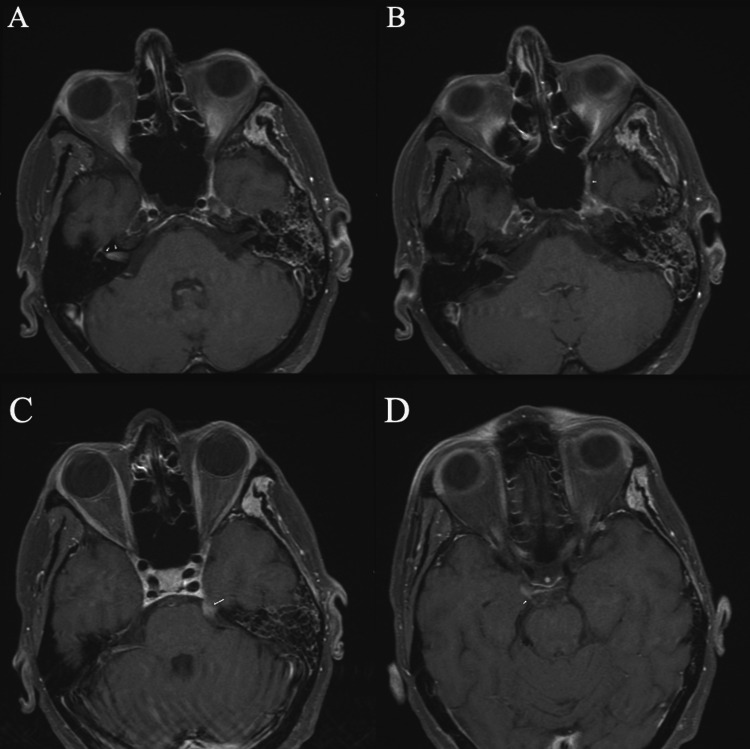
MRI of the brain with contrast revealing enhancement in multiple cranial nerves. (A) Linear post-contrast enhancement (*see arrows*) involving the right seventh and eighth nerve complex within the internal auditory canal, the basal turn of the right cochlea, and discontinuous enhancement of the right seventh cranial nerve up to its mastoid segment. (B) Linear post-contrast enhancement (*see arrow*) involving the V3 segment of the left trigeminal nerve. (C) Nodular enlargement with enhancement (*see arrow*) of the left trigeminal nerve in its cisternal segment. (D) Linear post-contrast enhancement (*see arrow*) involving the right third cranial nerve.
